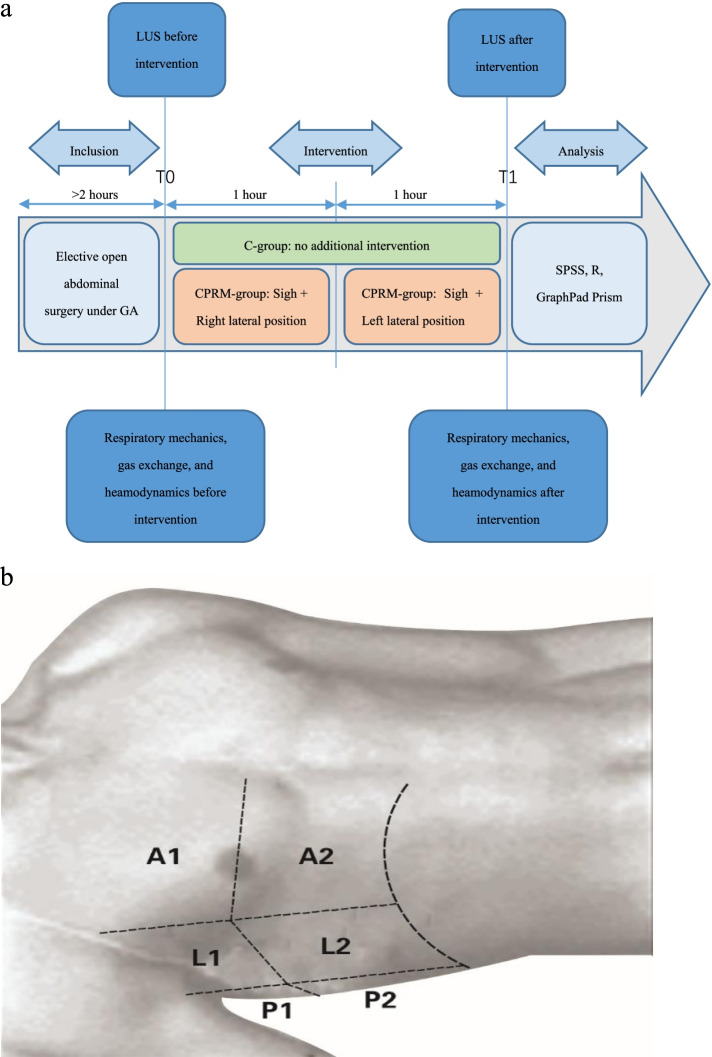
The study protocol. a LUS exams were performed for each patient before and after recruitment. T0, before recruitment; T1, after recruitment; C-group, control group; CPRM-group, combined physiological recruitment maneuver group; GA, general anesthesia; LUS, lung ultrasound. b Lung areas for LUS. A1, anterosuperior; A2, anteroinferior; L1, laterosuperior; L2 lateroinferior; P1, posterosuperior; P2, posteroinferior; LUS, lung ultrasound
